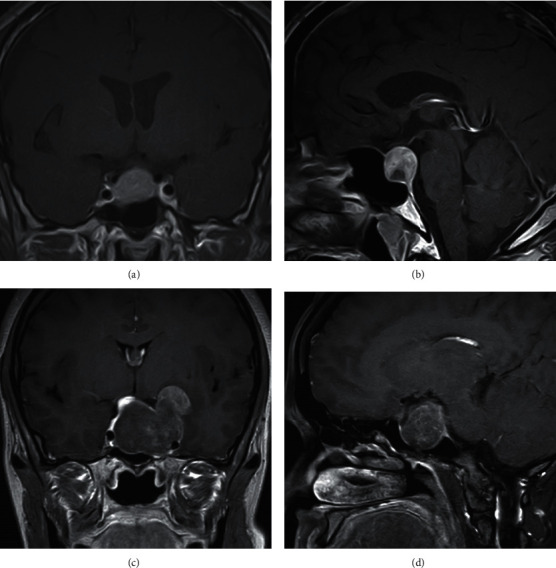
Enhanced magnetic resonance imaging of two patients with noninvasive pituitary adenoma (NIPA) and invasive pituitary adenoma (IPA). (a, b) Coronal and sagittal scans of the NIPA, respectively. (c, d) Coronal and sagittal scans of the IPA invading the left cavernous sinus and surrounding the internal carotid artery (Knosp classification grade IV).
